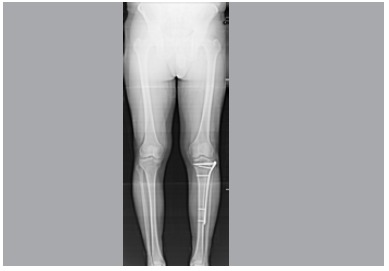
Ortho roentgenogram at last visit.
